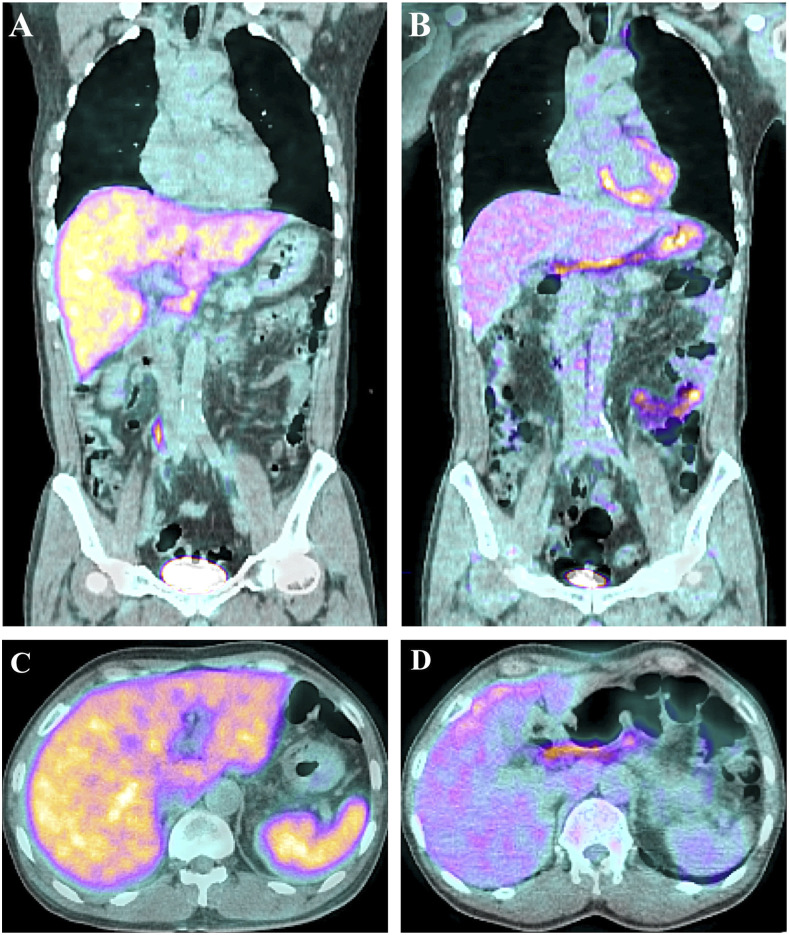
Coronal F-18 fluorodeoxyglucose positron emission tomography/computed tomography image before treatment (A) shows diffuse hypermetabolism in the liver, with hepatomegaly (AP diameter of the right hepatic lobe: 15.1 cm, SUVmax: 5.96, and SUVmean: 4.33). After treatment (B), the size and metabolism of the liver decreased to normal values (AP diameter of the right hepatic lobe: 13.7 cm, SUVmax: 2.53, and SUVmean: 1.77). An axial image before treatment (C) shows hypermetabolic splenomegaly (SUVmax: 5.59 and SUVmean: 4.46) that also normalized after treatment (SUVmax: 1.94 and SUVmean: 1.32) (D). Unlike for other infectious diseases, liver involvement was prominent. Hypermetabolic enlarged lymph nodes in the periportal and portocaval areas were also found on the initial image and returned to normal range after treatment. AP = anteroposterior; SUV = standardized uptake value. This figure appears in color at www.ajtmh.org.
